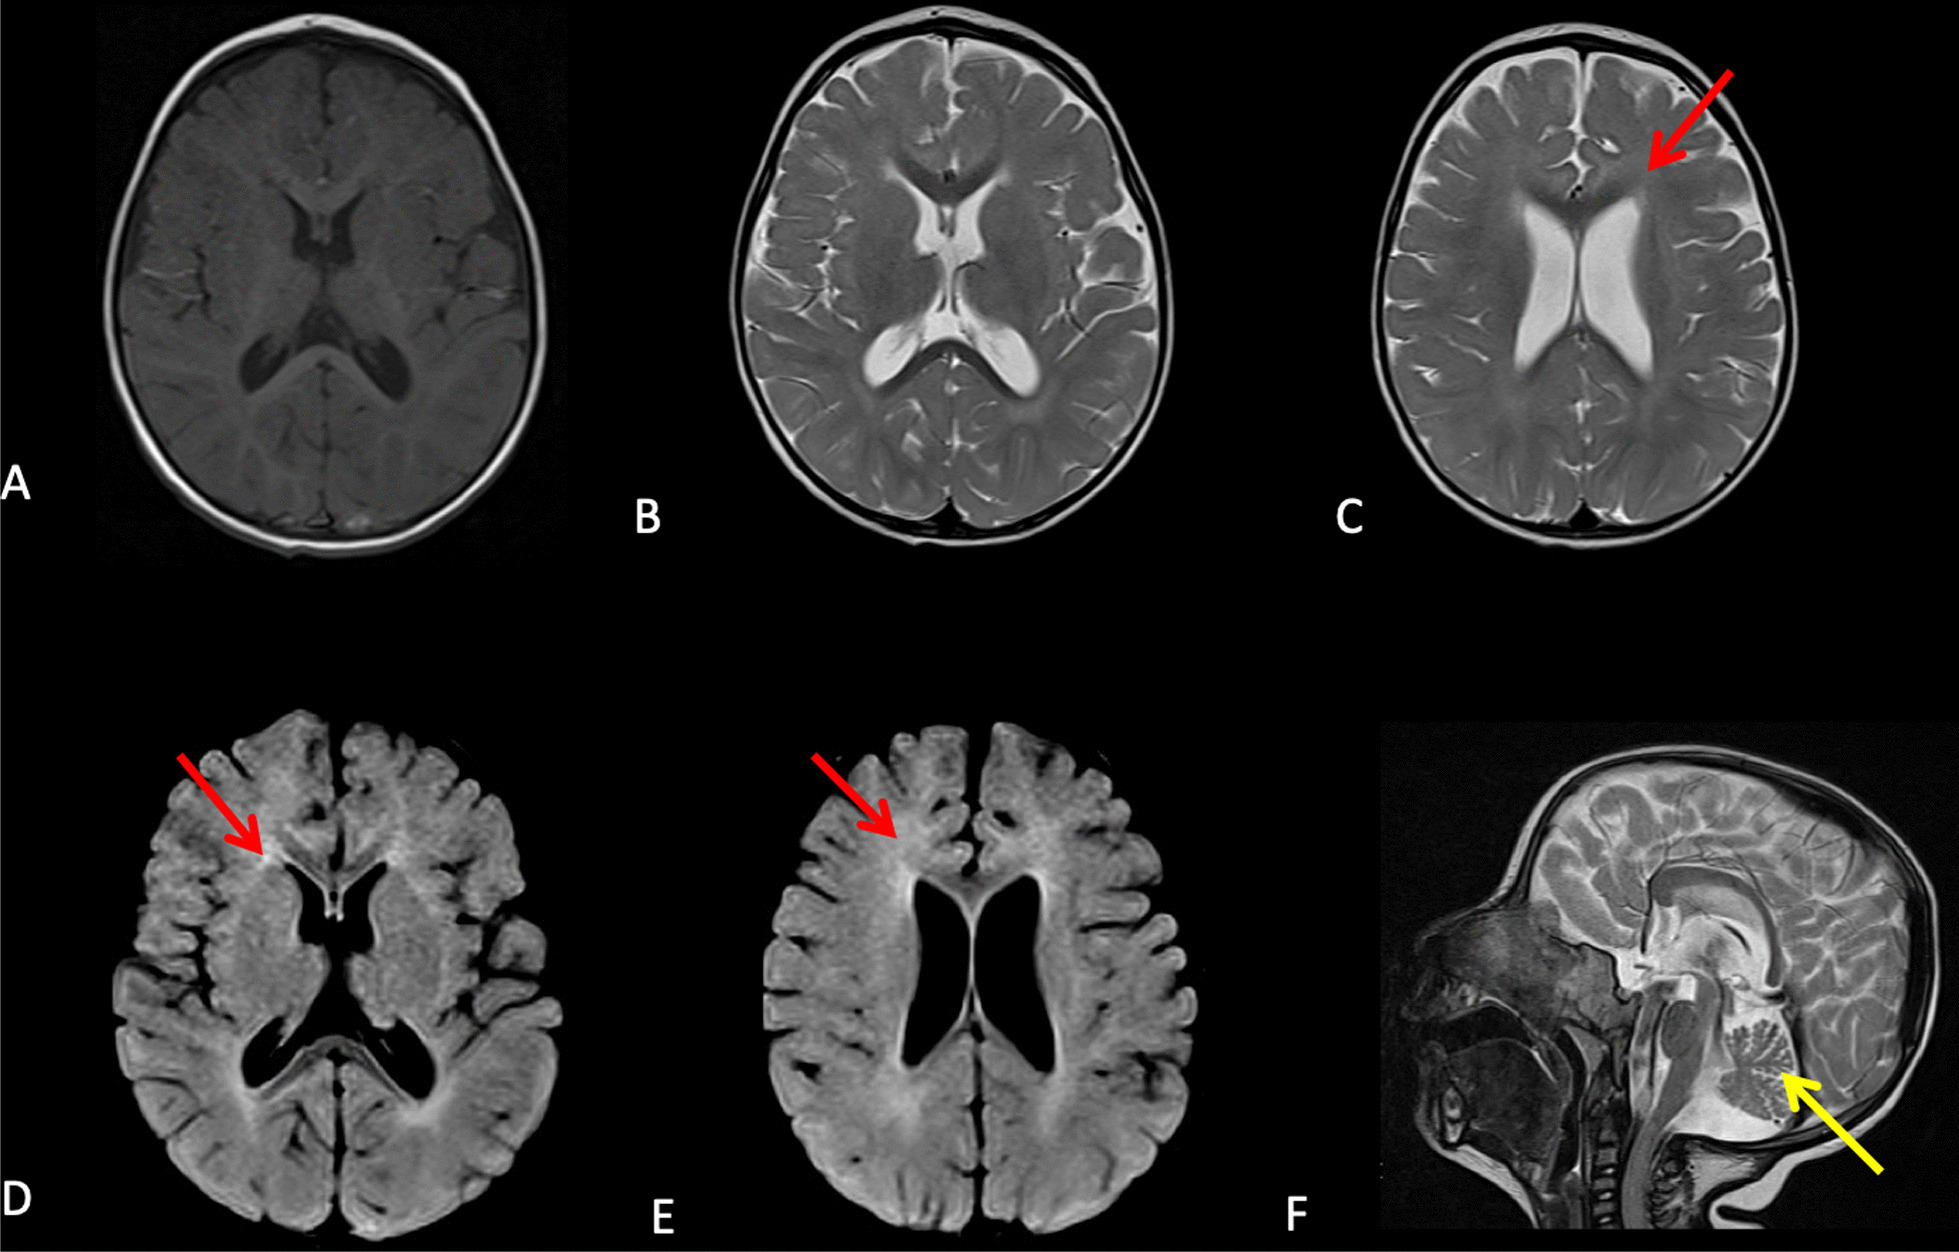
MRI image of CS7. A Axial T1 weighted-image, B, C axial T2 weighted-images, and D, E axial FLAIR weighted-images showing isointensity of periventricular white matter on T1, and hyperintensity on T2. FLAIR is suggestive of hypomyelinating leukodystrophy (red arrows). F Sagittal T2 weighted-image showing cerebellar atrophy (yellow arrow)
